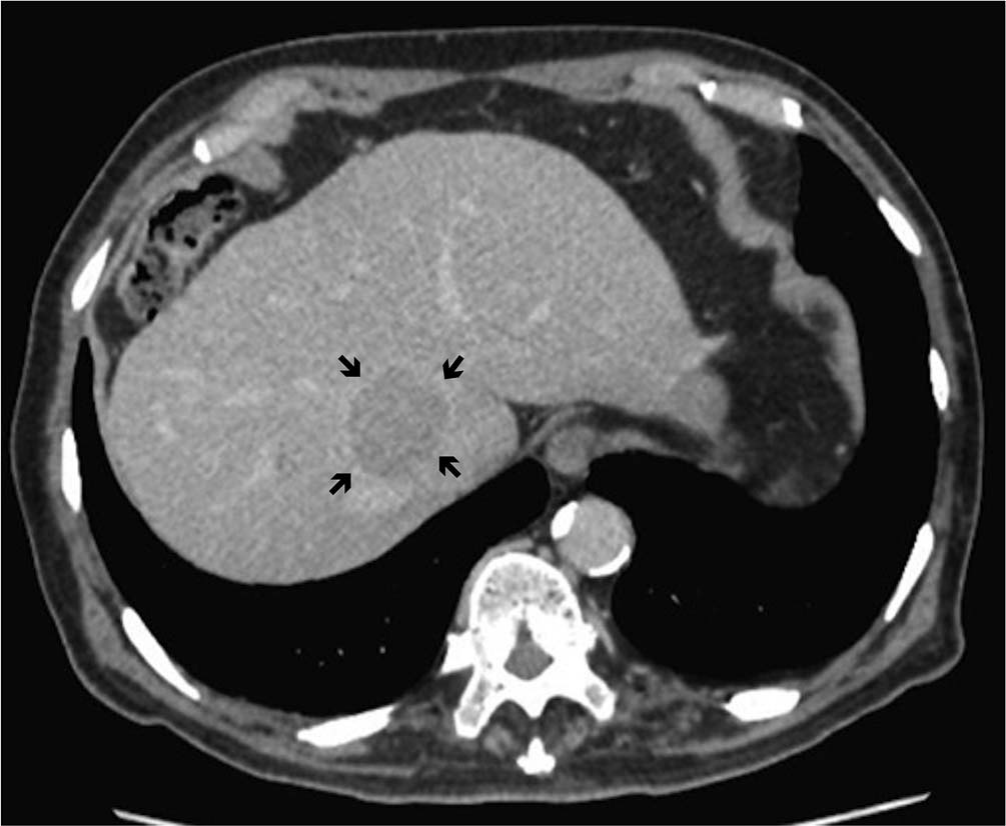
Dynamic computed tomography of the liver. Portal phase of dynamic CT shows a low-density area abutting the RHV, indicating hepatocellular carcinoma located in the caudate lobe (arrows).
